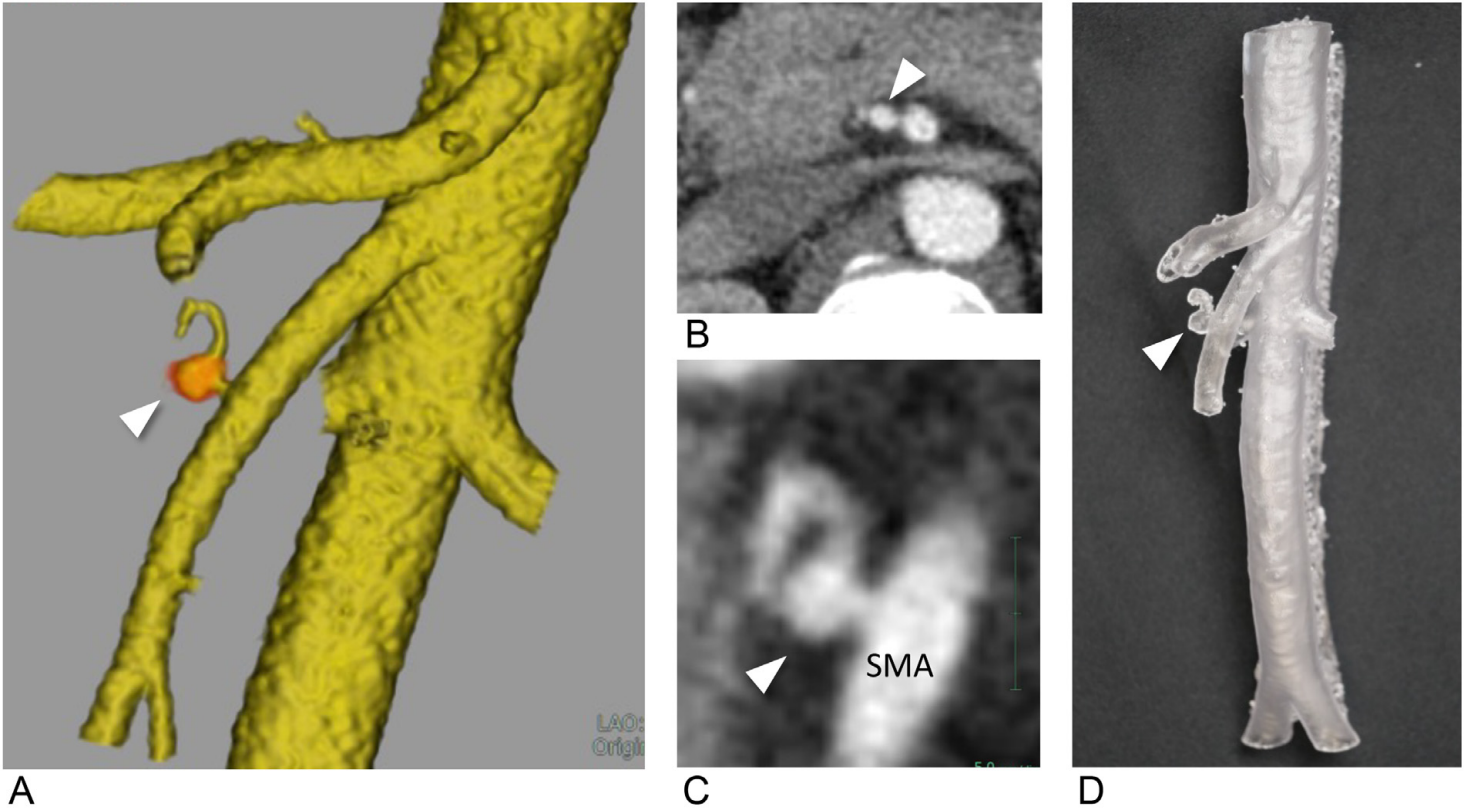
A 40-year-old female with a dorsal pancreatic artery aneurysm (Case 1). A 5 × 5 mm saccular aneurysm (white arrowhead) at the dorsal pancreatic artery originated from the superior mesenteric artery (SMA). Performing the filling coils without migrating from the SMA was challenging. (A) Volume rendering of computed tomography (CT) angiography showing a 5 × 5 mm saccular aneurysm (white arrowhead), (B) (C) CT angiography showing a 5 × 5 mm saccular aneurysm (white arrowhead), short neck to the SMA. (D) Transparent and flexible patient-specific hollow vascular models with dorsal pancreatic artery aneurysm (white arrowhead).
